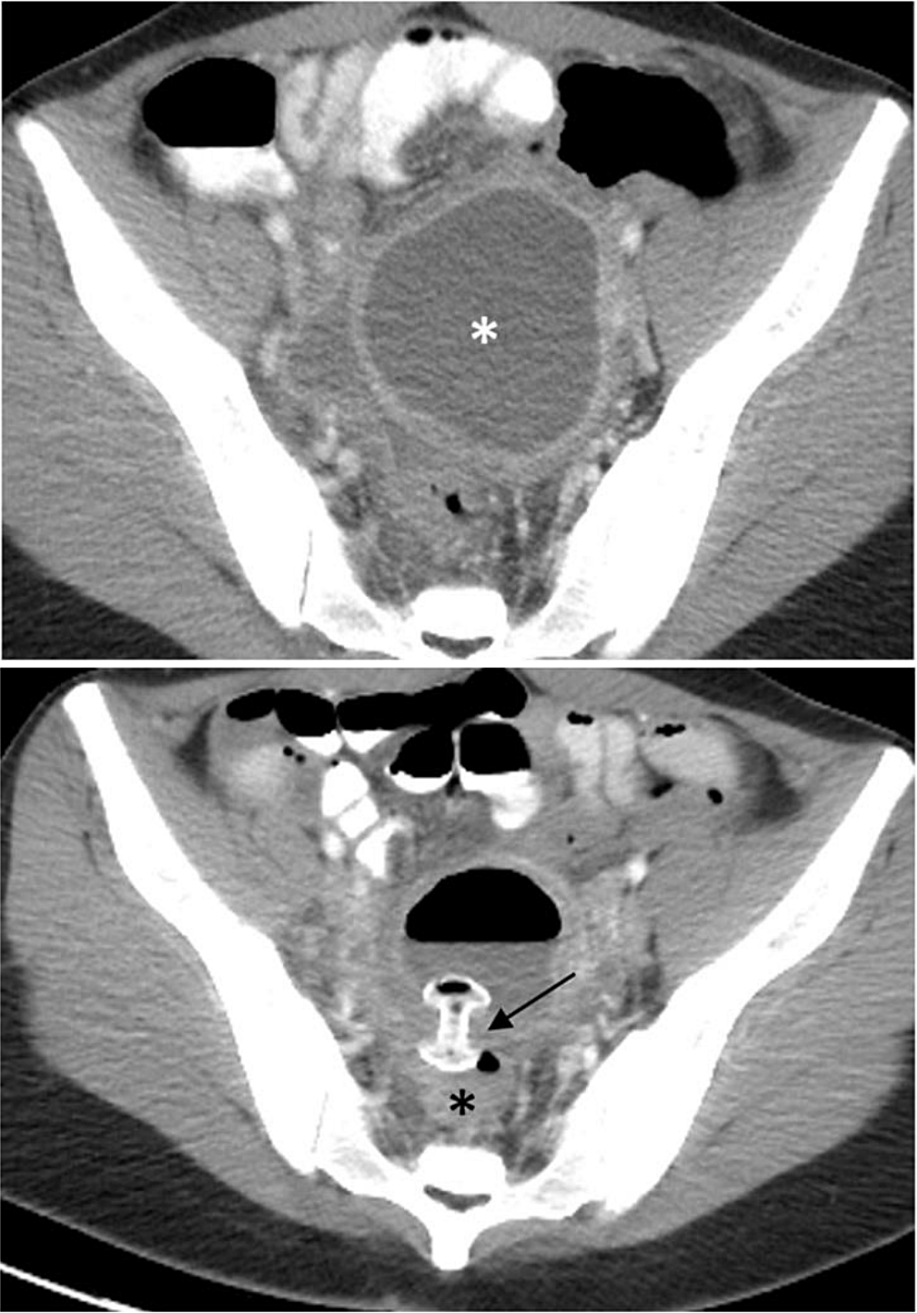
26 year-old female presented with ruptured tubo-ovarian abscess that was inaccessible by IR drainage. A. Contrast-enhanced axial CT image demonstrates ruptured tubo-ovarian abscess (white asterisk). B. Contrast-enhanced axial CT image demonstrates successful interval drainage into rectum (black asterisk) via LAMS (black arrow)
